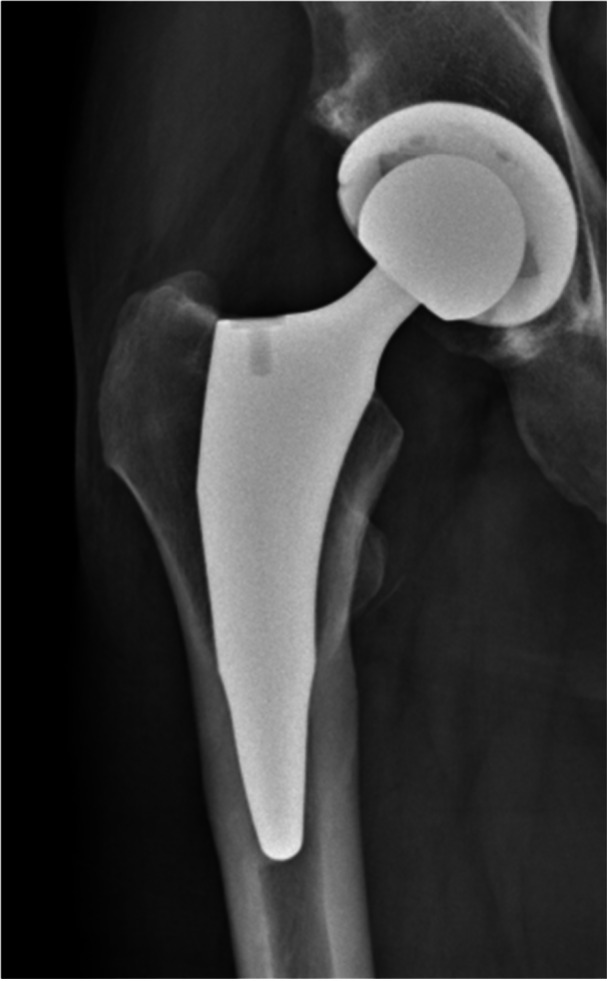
Two‐and‐a‐half‐year radiographic follow‐up showing cortical hypertrophy involving Gruen Zones 3–5.
